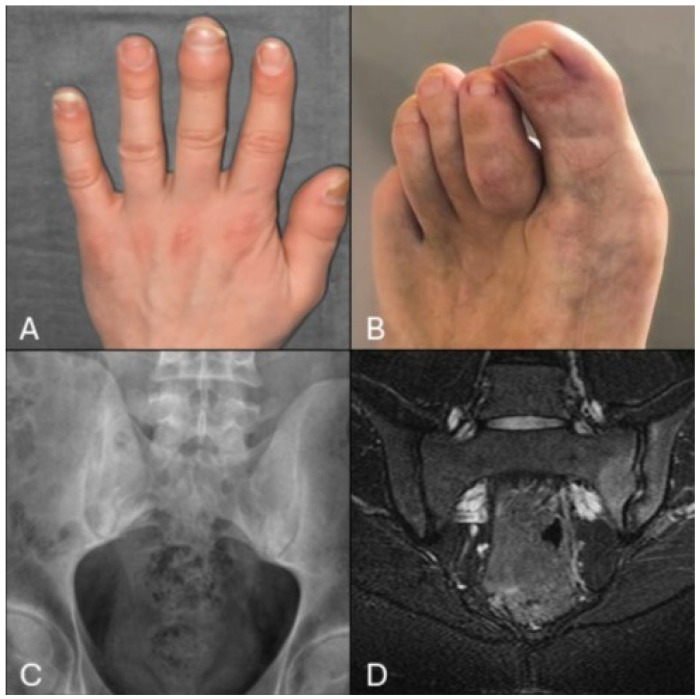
Typical clinical picture of patients with IBD-SpA: (A) distal interphalangeal arthritis of the 3rd finger; (B) dactylitis of the 2nd toe, often referred to as “sausage digit”; (C) plain radiograph of the pelvis showing sacroiliac joints sclerosis; (D) MRI of the pelvis revealing intraspongious edema of the left sacroiliac joint, a key feature of axSpA.
